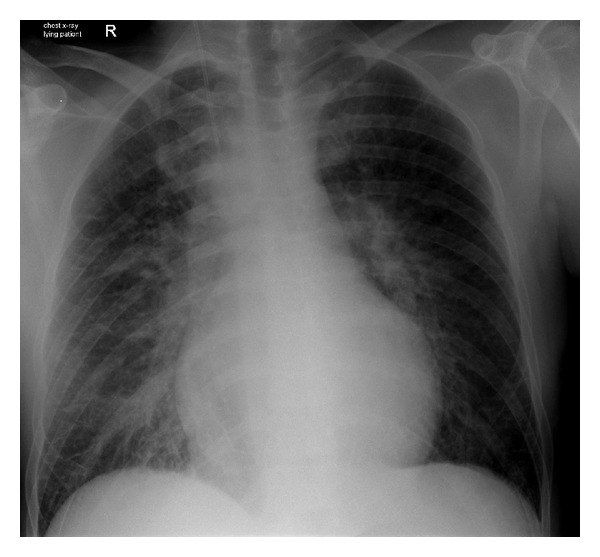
Chest X-ray of the lying patient in the a.p. path of ray. The congestion of the pulmonary veins reflects the cardiac shock and mild pulmonary edema. A meso-/dextrocardia with a dilated heart silhouette is the prominent finding.
